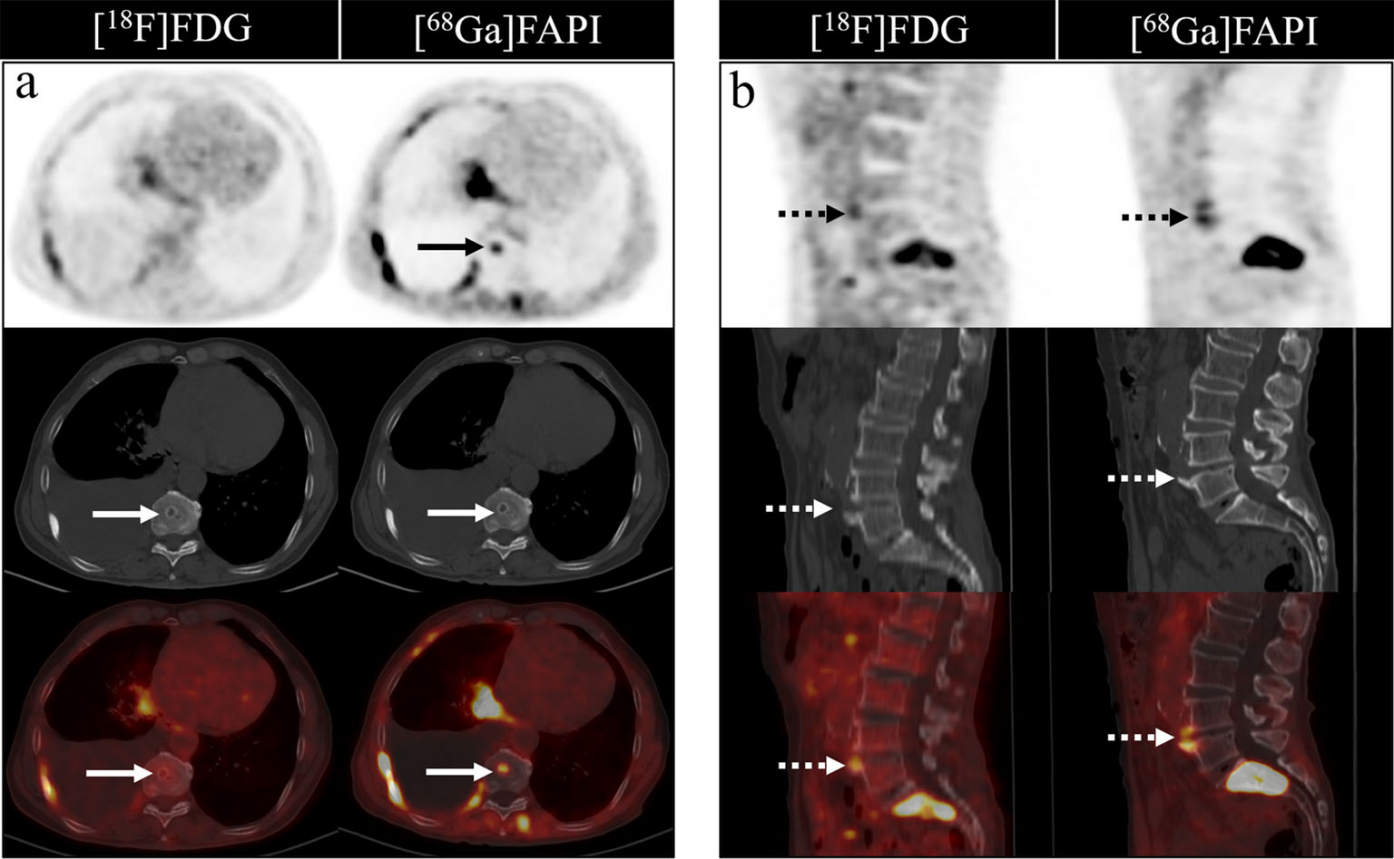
Characteristic findings of Schmorl Node (A) and degenerative osteophyte (B). The Schmorl Node in the patient with lung cancer (A) shows tracer uptake on the [^68^Ga]Ga-DOTA-FAPI-04 PET/CT, while no uptake on the [^18^F]FDG PET/CT. The degenerative osteophyte in the patient with lung cancer (B) shows tracer accumulation on [^18^F]FDG and [^68^Ga]Ga-DOTA-FAPI-04 PET/CT.
